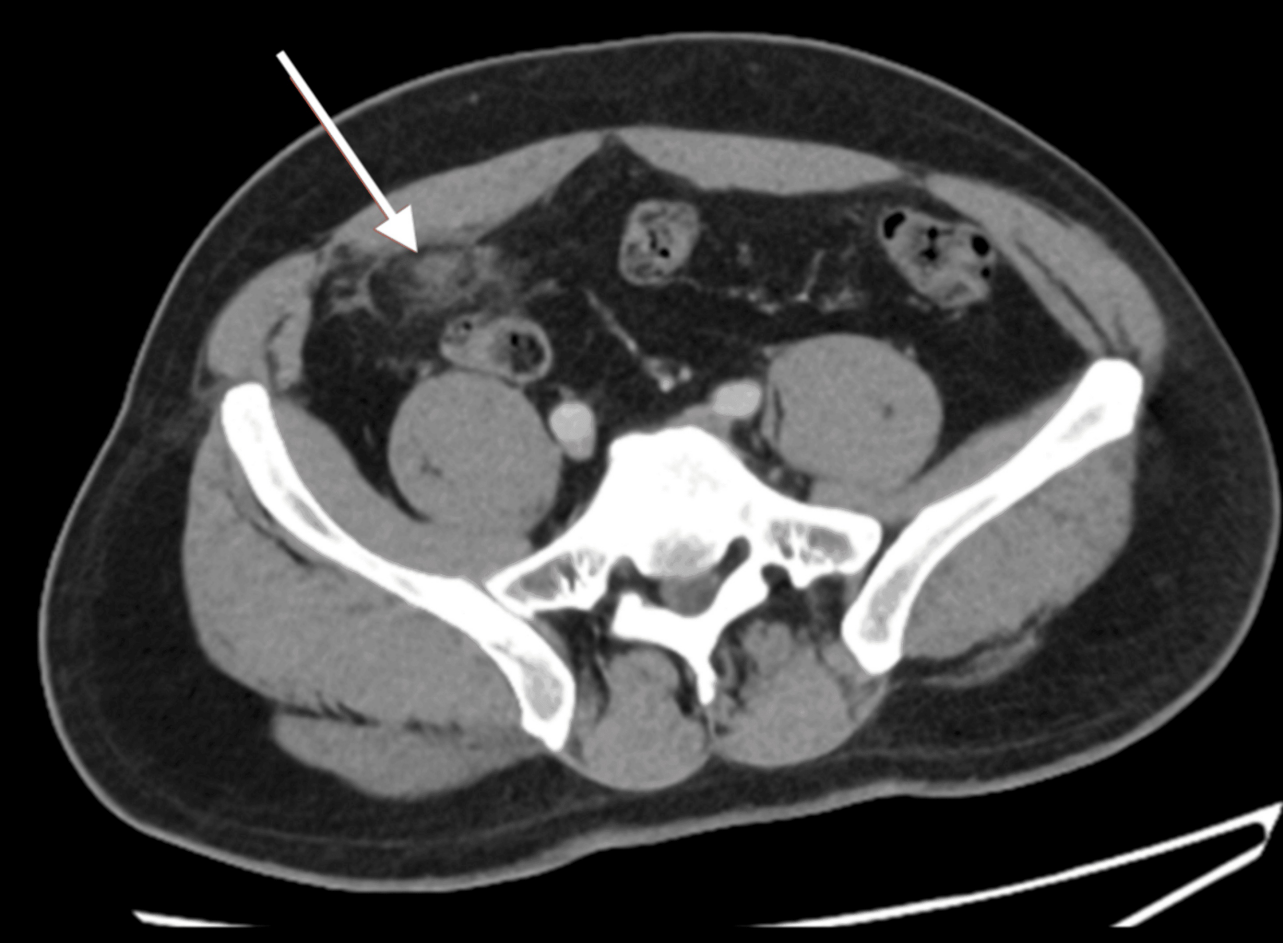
Axial CT image showing a focal area of fat with hyperattenuating streaks and surrounding stranding (arrow), with associated peritoneal thickening and mild vessel dilation.
